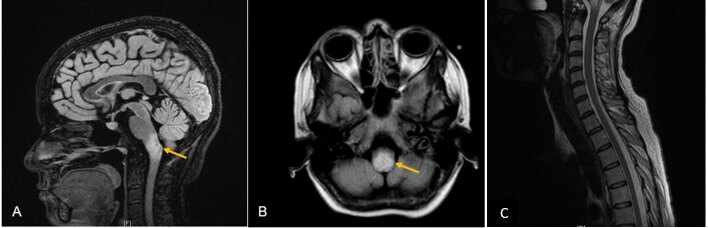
MRI demonstrating isolated medullary involvement. (A) Sagittal T2-FLAIR image showing a focal hyperintense lesion in the dorsal medulla (arrow), with no signal abnormality in the cervicomedullary junction. (B) Axial T2-FLAIR image demonstrating the corresponding medullary hyperintensity (arrow). (C) Sagittal T2-weighted image of the cervico-dorsal spinal cord showing no signal abnormality.
